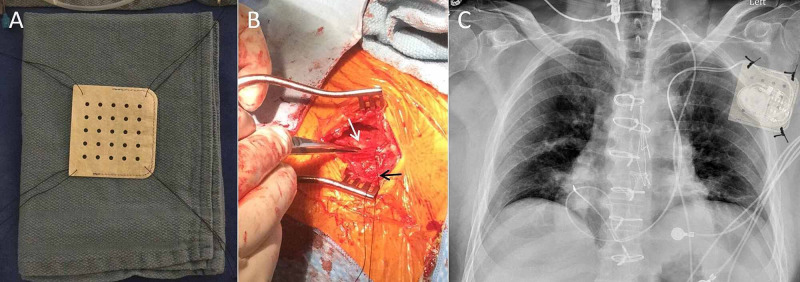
CanGaroo® envelope pocket securement technique. (A) Silk sutures have been placed in all four corners of the envelope to demonstrate securement options. (B) The PPM has been placed in the CanGaroo® envelope (white arrow) and a silk suture (black arrow) has been placed in one corner. (C) Anterior-posterior chest X-ray of a dual-chamber PPM via a left persistent SVC with an image overlay of the CanGaroo® envelope and anchoring sutures representative of a three-point securement technique. PPM, pacemaker; SVC, superior vena cava
